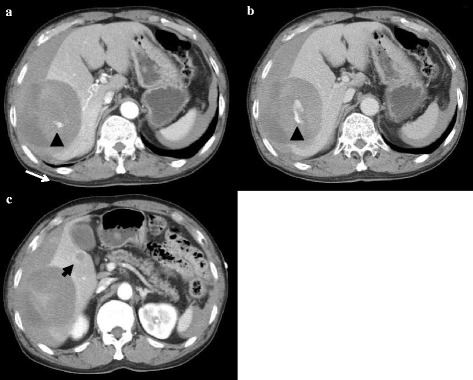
Contrast-enhanced computed tomography scan showed a hypo-enhancing tumor (98 × 81 mm) in the posterior segment of the liver with intratumoral extravasation on arterial phase (a) and on delayed phase (b). A daughter tumor (12 × 10 mm) in segment 5 of the liver was also detected (c)
